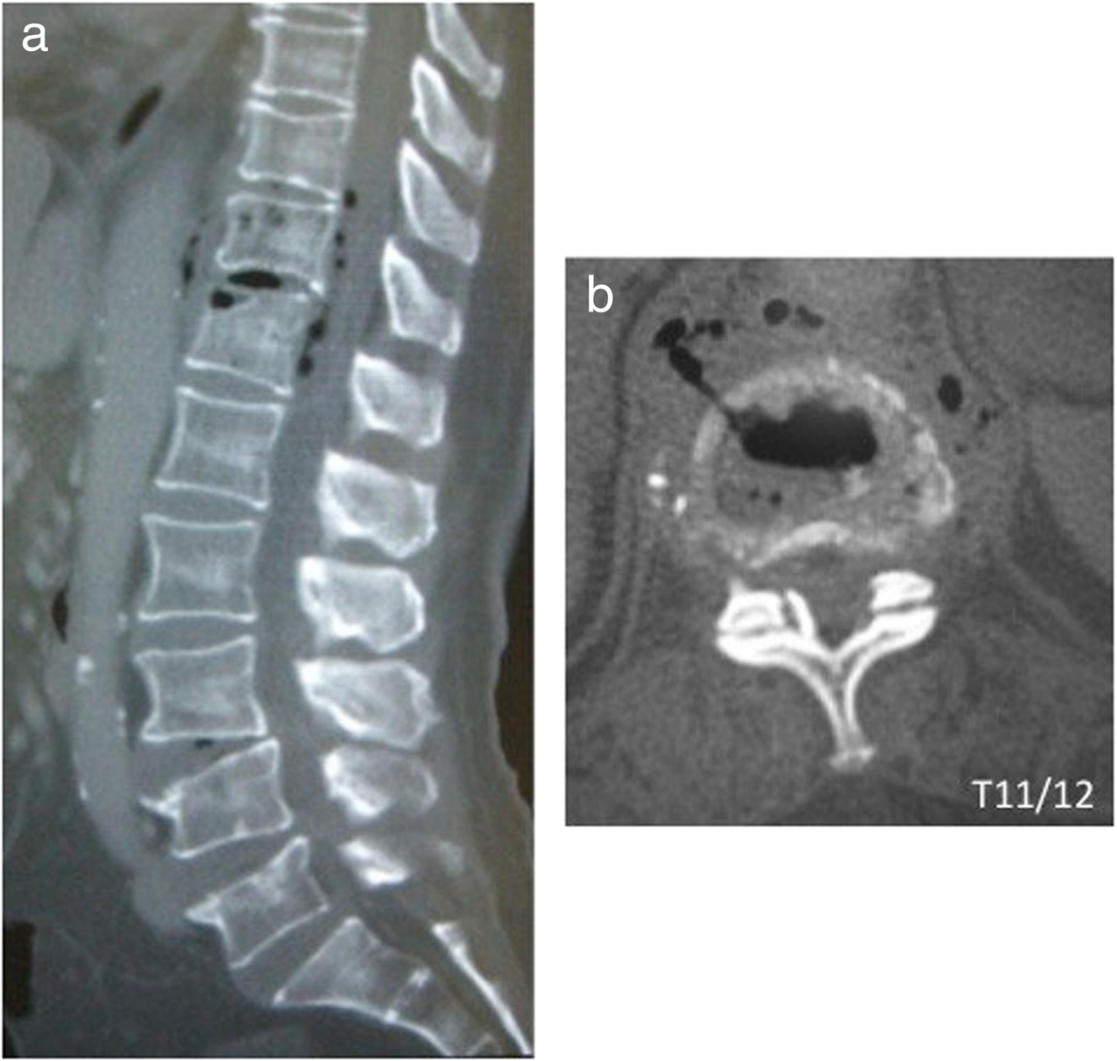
Computed tomography sagittal and axial images on initial admission. (a) Soft tissue swelling anterolaterally at intervertebral disc level T11/12 (b) and a gas-containing epidural abscess are seen.
